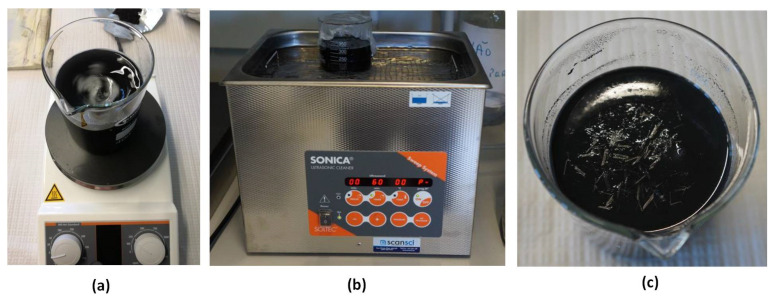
Preparation of aqueous suspensions: (a) magnetic stirring, (b) ultrasonication and (c) the aqueous suspension containing CFs and MWCNTs.
